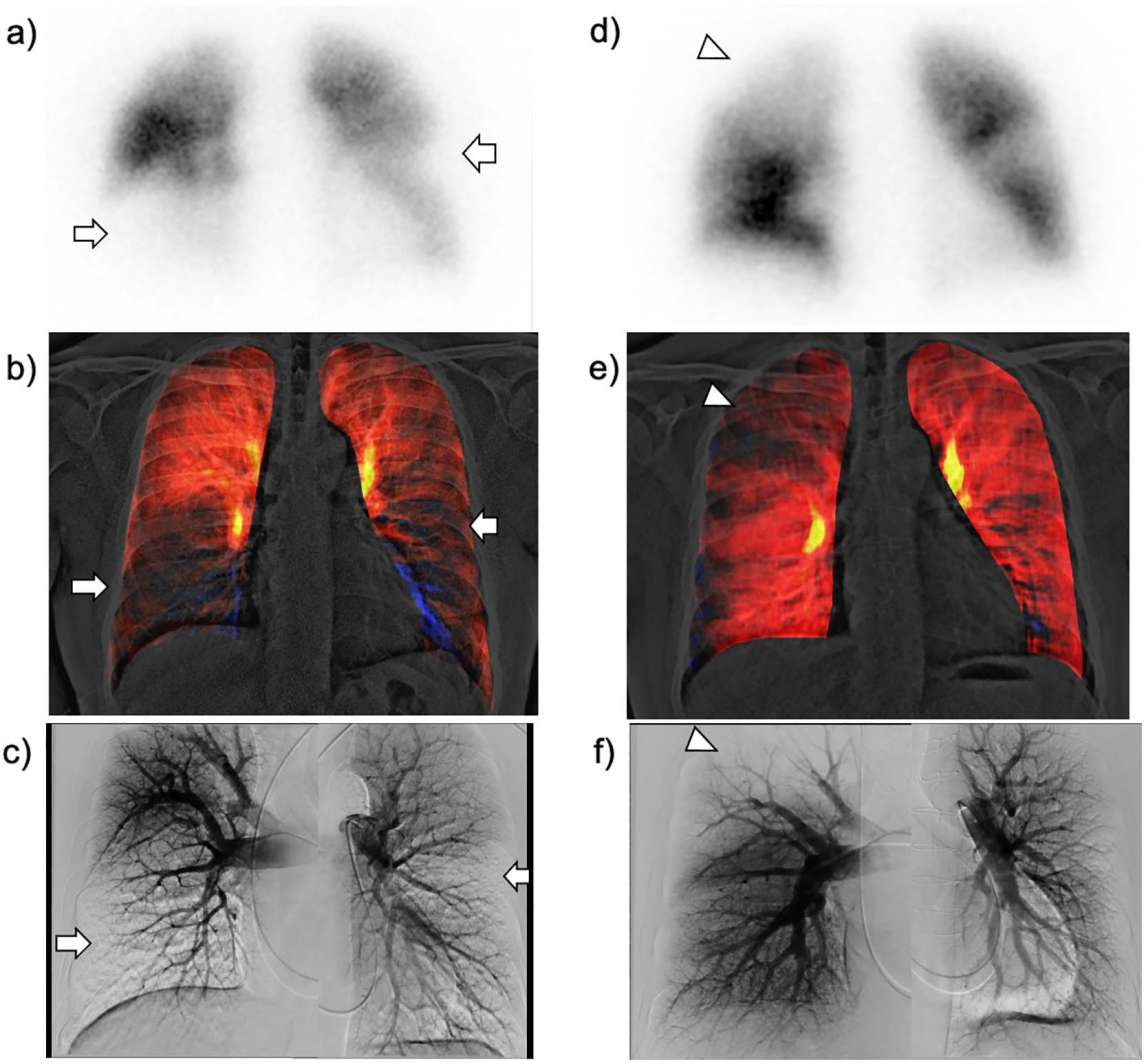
A 46-year-old man with chronic thromboembolic pulmonary hypertension before (a–c) and after (d–f) pulmonary endarterectomy (PEA). Tc-99 m macroaggregated albumin lung perfusion scintigraphy showing multiple perfusion defects in both the lungs (a, arrows). A lung perfusion image created from dynamic chest radiography (DCR) demonstrates lung perfusion defects (b), similar to lung perfusion scintigraphy and pulmonary angiography (a, c). After PEA, the lung perfusion image of DCR (e) demonstrates improvement in lung perfusion in the lower lungs, bilaterally, with a relative decrease in the right upper lung, which is the so-called vascular steal (d–f, arrowheads). *Reproduced with permission from Ref 27 (Yamasaki Y, et al. A novel pulmonary circulation imaging using dynamic digital radiography for chronic thromboembolic pulmonary hypertension. Eur Heart J. 2020;41(26):2506)
